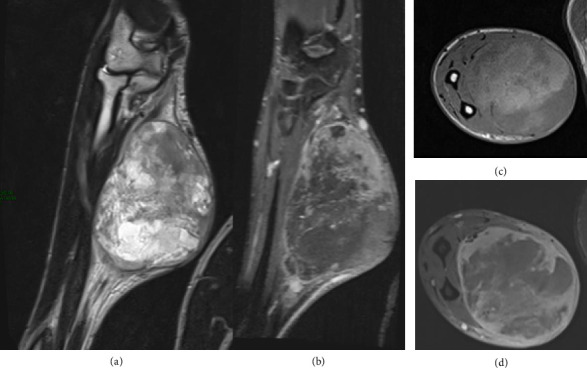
MRI images. A well-defined solid mass with regular margins located in the middle third of the medial compartment of the forearm. The lesion appears to originate along the course of the ulnar nerve, not clearly recognizable, involving the flexor muscles, both intra and interfascial. A thin plane of cleavage from the ulnar diaphysis is preserved. (a) Coronal T2 TSE; heterogeneous with a prevalent hyperintense component, due to a fluid component. (b) Coronal T1 postcontrast; the lesion shows heterogeneous postcontrast enhancement. (c) Axial T1 TSE; (d) Axial T1 postcontrast.
